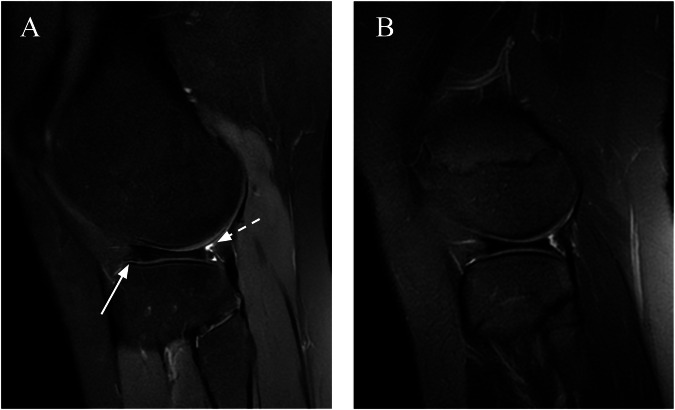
A 20-year-old woman underwent a follow-up examination of the DM. A Sagittal FS T2-W MR image of the right knee showing anterior bulging of the meniscal margin that was not evident in a previous exam (B) performed 5 years before. Note, the absence of the posterosuperior popliteomeniscal fascicles (dotted arrow) (Wrisberg type). These signs together suggest PRI
